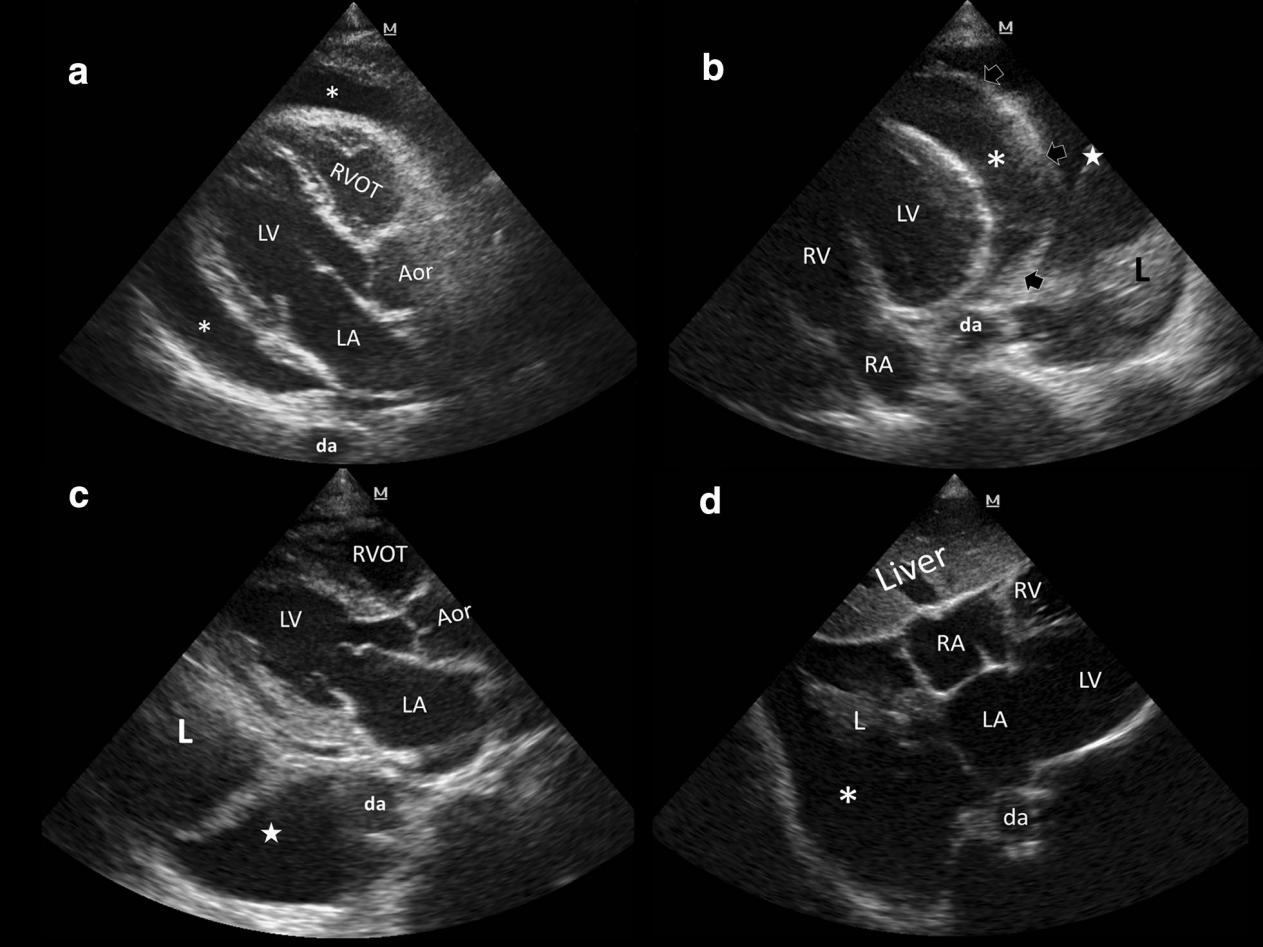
Pericardial versus pleural effusion. a Parasternal long axis view. Pericardial effusion (*asterisks*) tracks anterior to the descending aorta (da); *LV* left ventricle, *LA* left atrium, *RVOT* right ventricle outflow tract, *Aor* aortic root. b Apical 4-chamber view. Pericardial effusion (*asterisk*) tracks anterior to the descending aorta (da); pleural effusion (*white star*) tracks posterior and lateral to the da. *Arrows* pericardial delineation, *L* lung consolidation, *LV* left ventricle, *RV* right ventricle, *RA* right atrium. c Pleural effusion (*white star*) tracks posterior and lateral to the da; *L* lung consolidation, *LV* left ventricle, *LA* left atrium, *RVOT* right ventricle outflow tract, *Aor* aortic root. d Right pleural effusion (*asterisk*) extending into the bare area of the liver, besides the heart in subcostal 4-chamber view; *L* lung consolidation, *RA* right atrium, *RV* right ventricle, *LA* left atrium, *LV* left ventricle, *da* descending aortaPEF is always present in dependent segments (posterior wall, lateral wall, inferior wall).Checking diastolic collapse of cardiac chambers that is a sign of hemodynamically significant PEF: characteristically, the right ventricle outflow tract and the right atrium (RA) are the first parts of the cardiac chambers that collapse under the external pressure of PEF, followed next by the right ventricle (RV) with further increase in the pericardial pressure; diastolic collapse of the left atrium (LA) and left ventricle (LV) can be observed only in the most extreme cases [9, 10]. Right chambers will be fairly resistant to collapse in severe pulmonary hypertension, when abnormal high intracavitary pressure is opposed to the high intra-pericardial pressure [11]. Of note, diastolic collapse of both atriums in significant PEF occurs in ventricular systole (i.e., atrial diastole), and thus one can determine its presence when the atrioventricular valves are closed. This allow for distinction between diastolic collapses in PEF from the normal atrial systole which occurs when the atrioventricular valves are opened.Checking dilated (more than 2 cm diameter in long axis) and reduced or absent collapsibility (less than 20% collapse) of the inferior vena cava (tamponade) in spontaneously breathing patients [9, 10].
